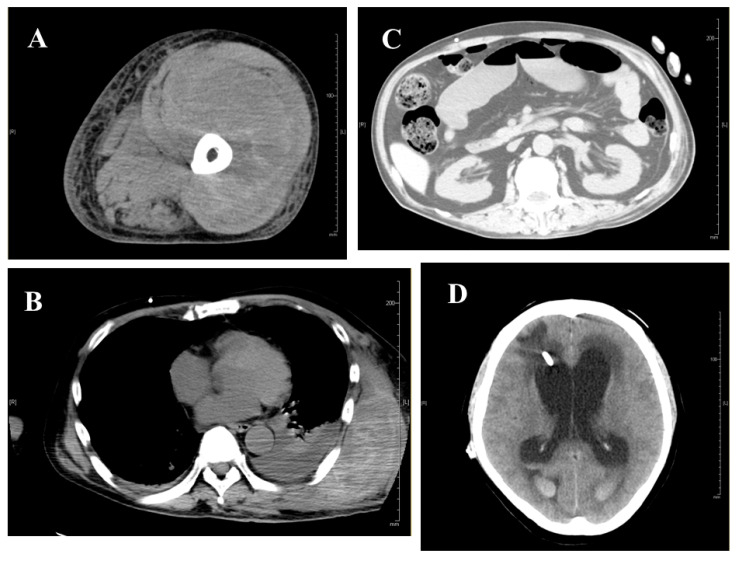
(A) CT image of a left femoral hematoma at disease onset. (B) CT findings of subcutaneous hematoma and intrathoracic hemorrhage on day 56 after admission. (C) CT image demonstrating strangulated ileus complicated by small bowel perforation, requiring laparotomy on day 80 after admission. (D) CT findings of intracranial hemorrhage on day 102 after admission.
